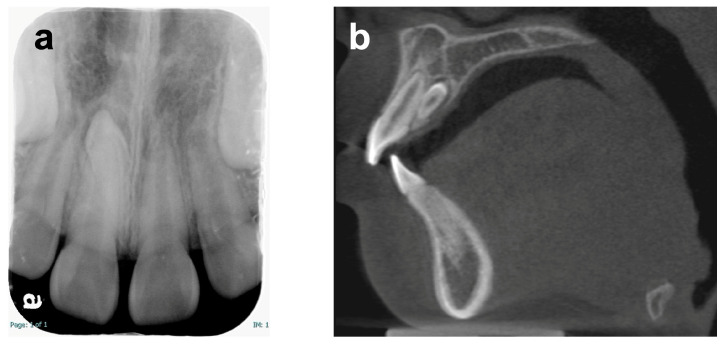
Dental radiography and cone-beam computed tomography imaging (age 8 years 9 months). (a) Dental radiograph; (b) Sagittal computed tomography image.
